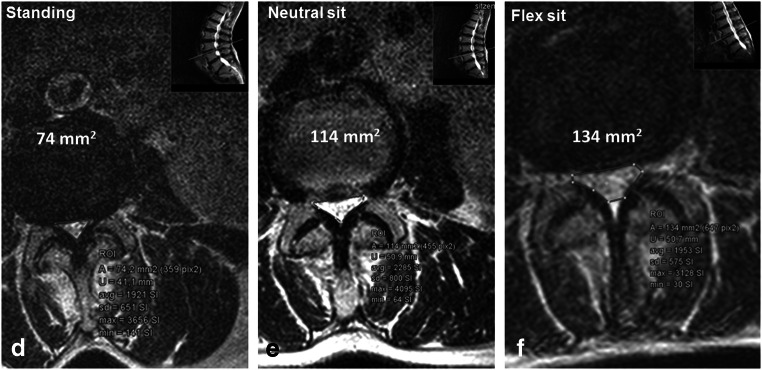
Axial T2-weighted images of the same patient at the stenotic level L3/L4 presenting the measurement of the DSCA in d standing, e neutral sitting and f flexed sitting positions
